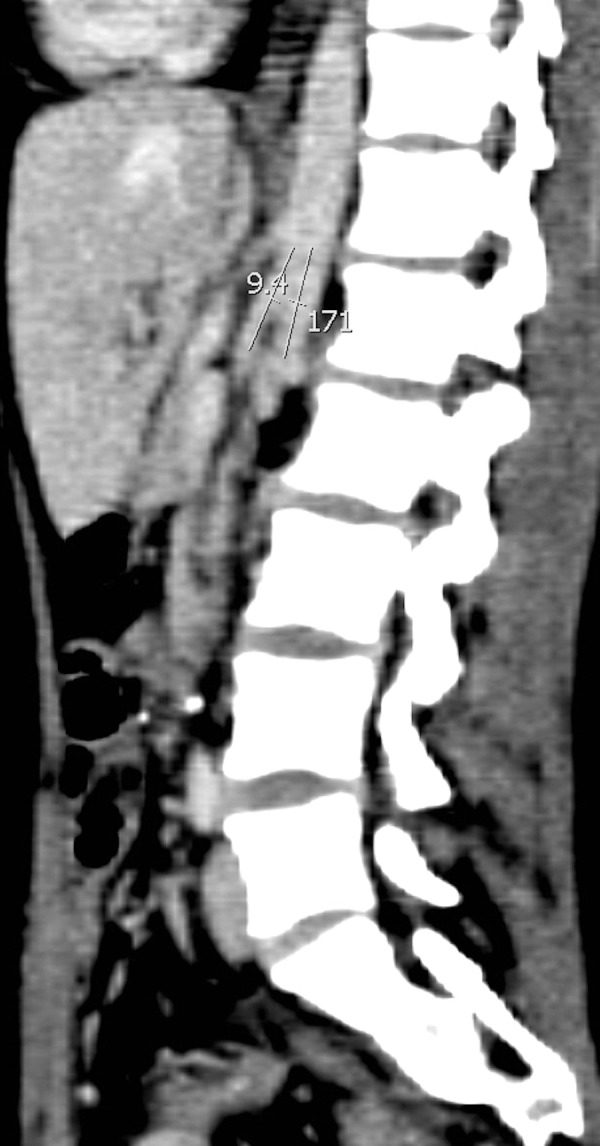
Contrast-enhanced sagittal CT image demonstrates a narrow aortomesenteric angle of 9° (calipers) (normal > 22°).
